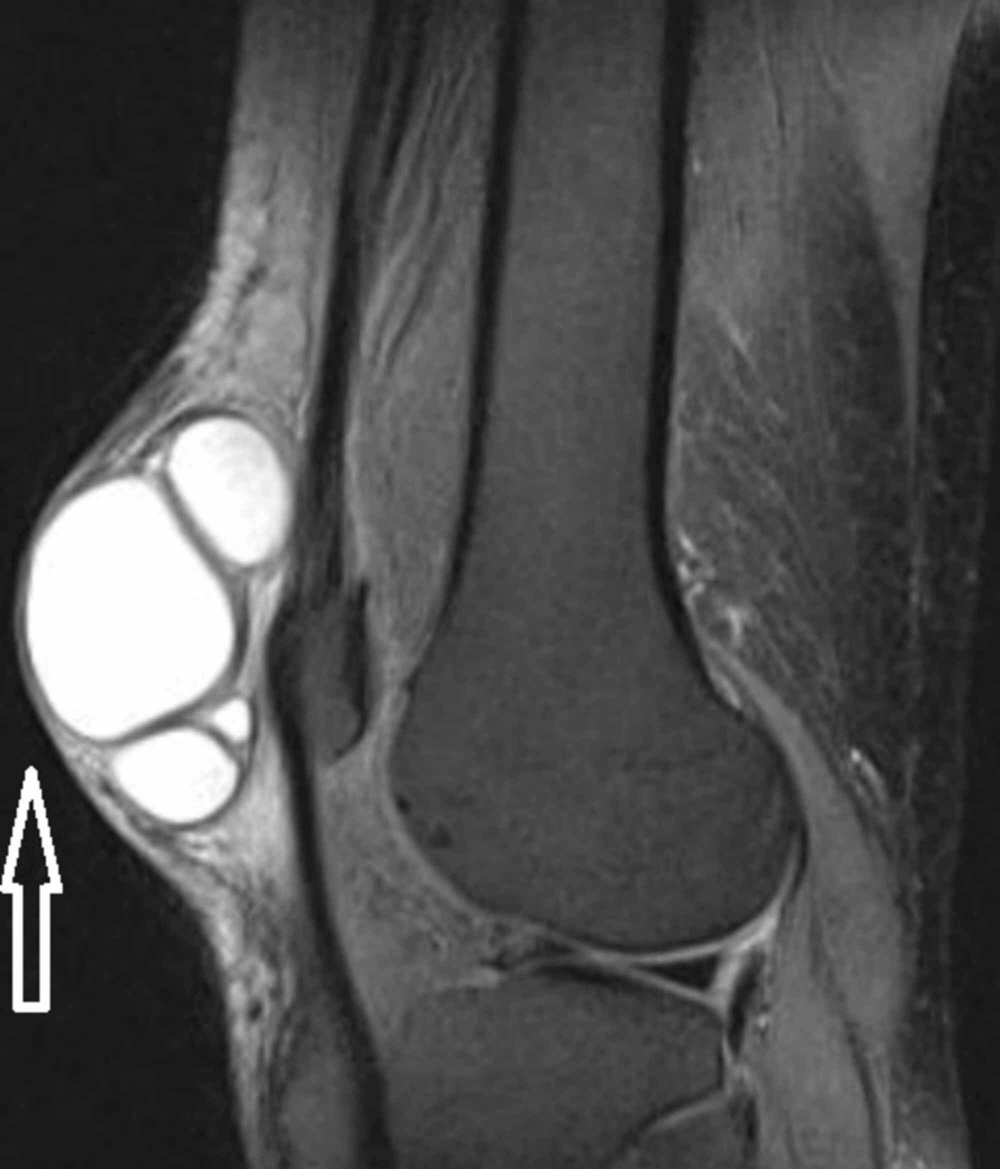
MRI of the right knee (coronal view) showing hypoechoic cystic loculated lesion in subcutaneous plane (arrow)
